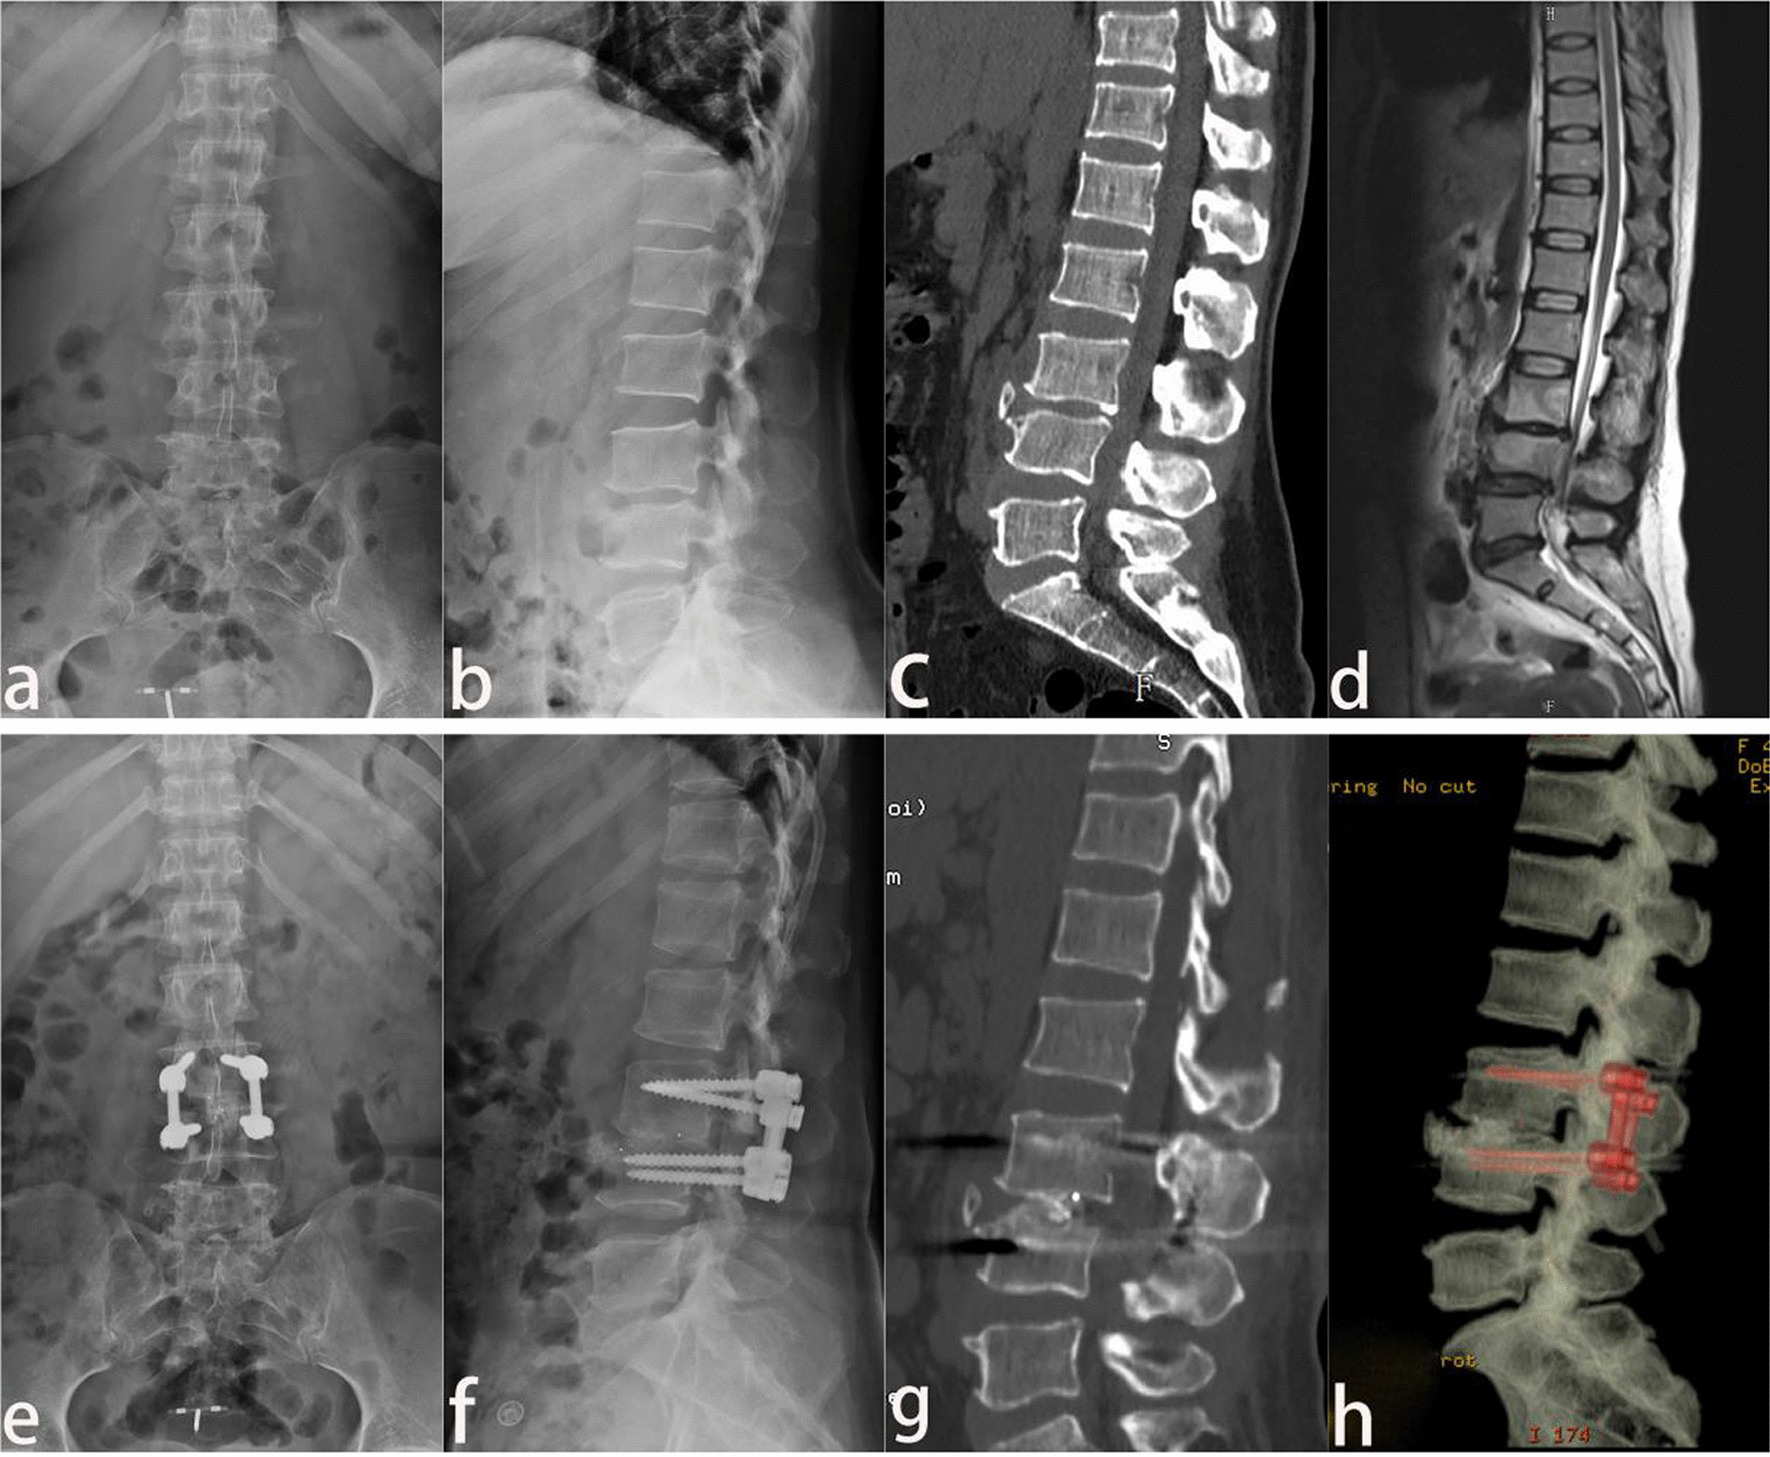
A 44-year-old female with lumbosacral Brucella spondylitis. a–d The lesion of the lumbosacral spine (L3, L4) was shown by the preoperative positive and lateral X-ray, CT sagittal reconstruction, and MRI. e, f The vertebral body was fixed firmly by the screw at 3 postoperative months, which was presented by X-ray. g, h CT sagittal and three-dimensional reconstruction demonstrated that the lesion was removed completely, and the internal fixation was stable without recurrence of the lesion at 6 postoperative months
